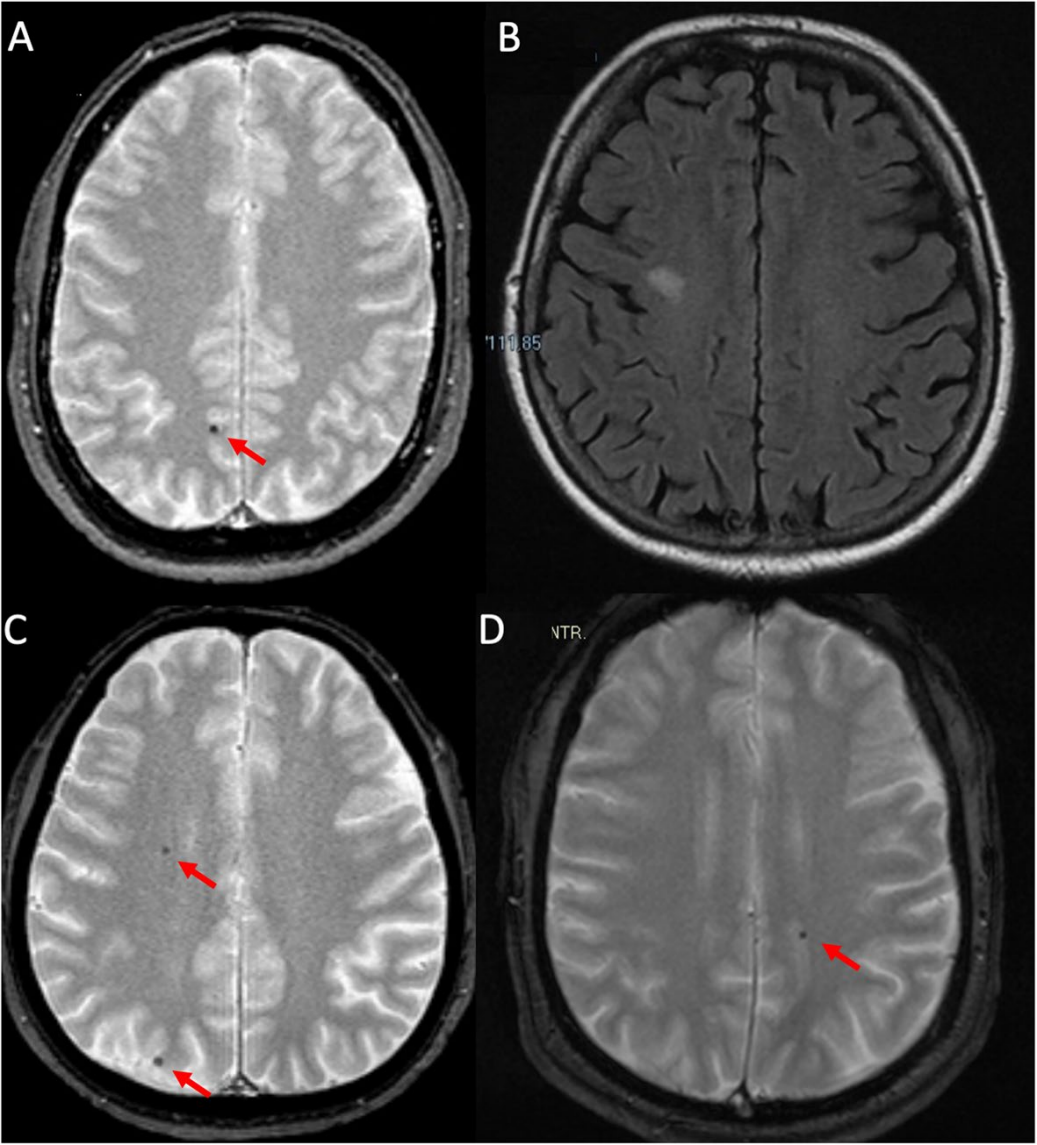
Brain-MRIs. Figure 2 shows brain MRI of the four patients included in this case series. Patient 1 (A): brain MRI revealed small microbleeds (MBs), the greater of which was localized in right precuneal region (red arrow). Patient 2 (B): brain MRI revealed slight signs of chronic ischemic vascular suffering. Patient 3 (C): brain MRI showed small MBs in the right frontal region and right occipital pole (red arrows). Patient 4 (D): brain MRI revealed small MB in the left post-rolandic parietal region (red arrow). For other MRI findings see Tab 1
